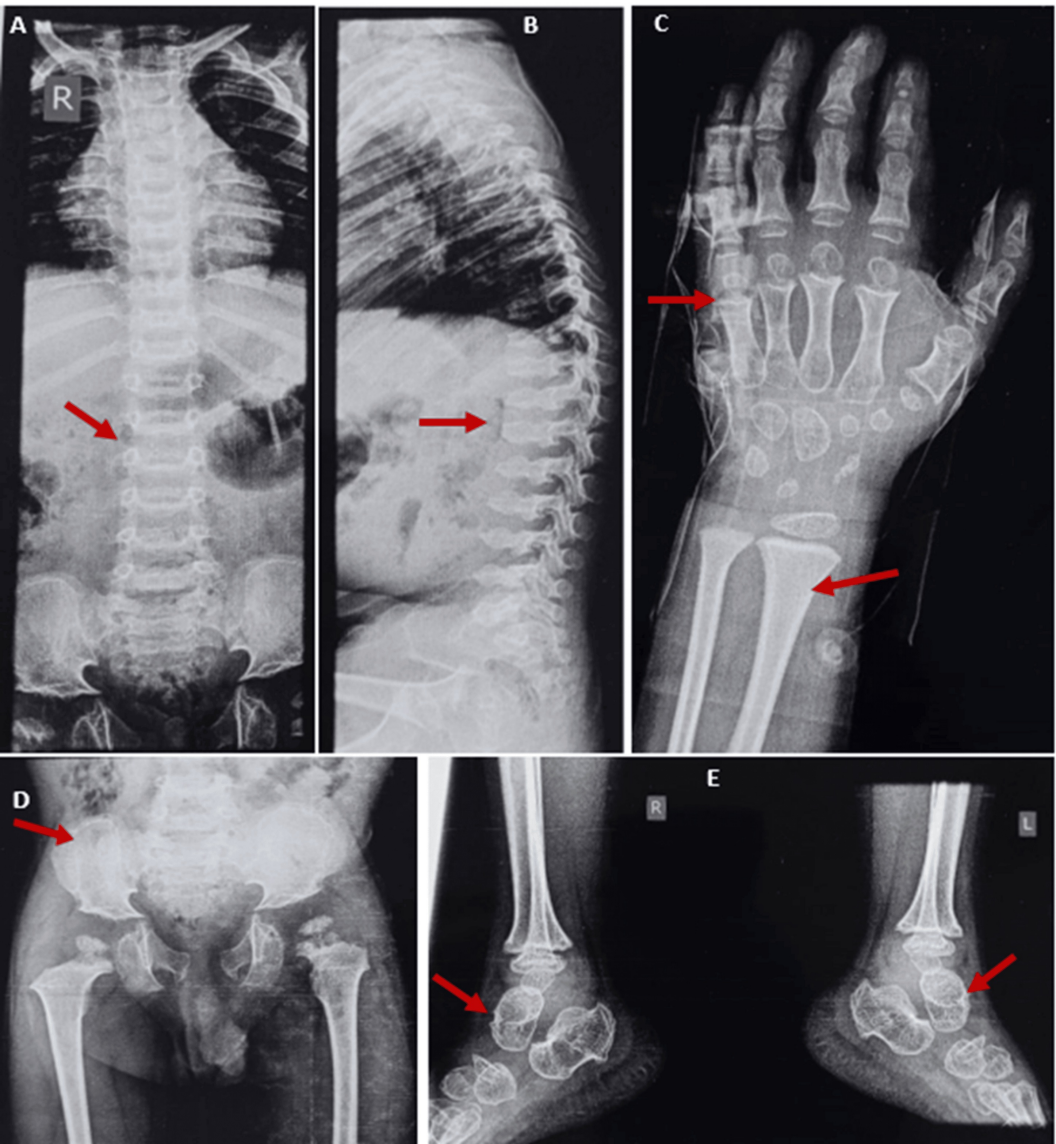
X-ray images (A,B) X-ray spine anteroposterior and lateral views showing scoliosis, anterior beaking, and double-humped thoracic and lumbar vertebral bodies. (C) X-ray hand and wrist anteroposterior view showing short and thick metacarpals, bullet-shaped phalanges, and dysplastic radial and ulnar epiphyses. (D) X-ray of the pelvis showing the non-visualization of the lower sacral vertebrae, an abnormal pelvic inlet, widening of the pubic symphysis, along with a lacy pattern of the iliac crest, a short femoral neck, and irregular femoral epiphysis and metaphysis. (E) X-ray of the bilateral foot and ankle joint revealed a congenital vertical talus
